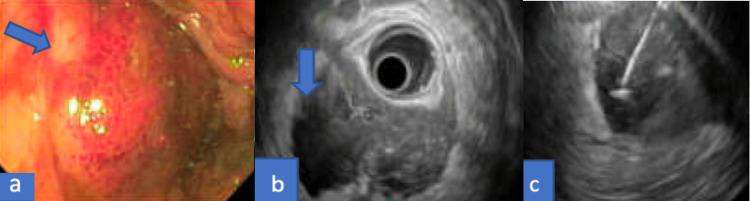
Endoscopic ultrasound (EUS) demonstrated a 4.7 cm x 3.8 cm non-circumferential mass located at the posterior rectal wall: (a) endoscopic image, (b) EUS image, (c) fine-needle aspiration (FNA) of the mass. EUS findings suggest a tumor originating from the muscularis propria with invasion into the perirectal fat, suggestive of a gastrointestinal stromal tumor (GIST).
